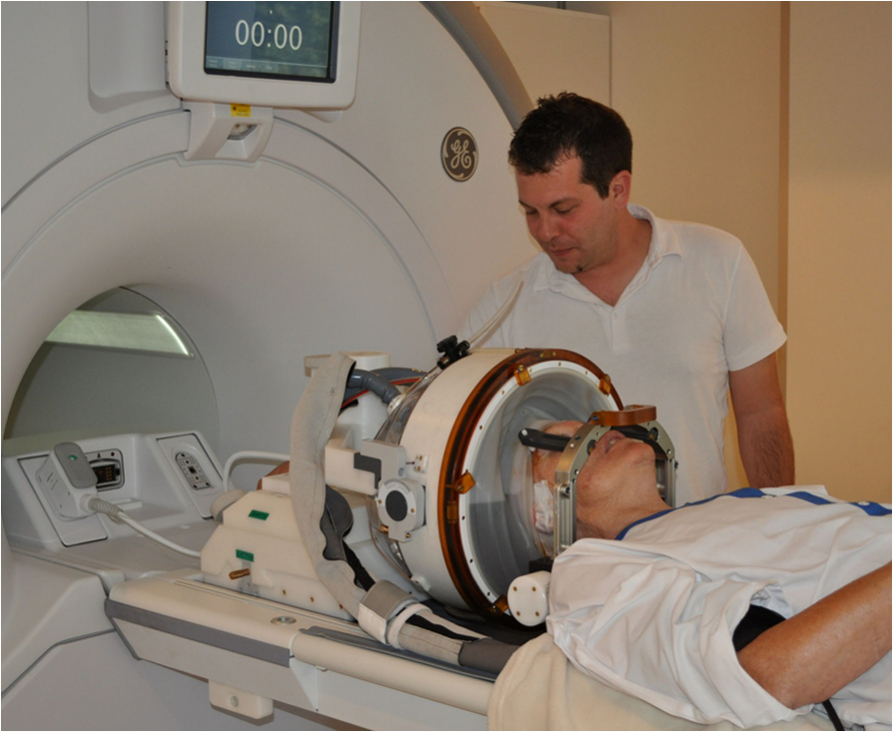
Intra-operative patient setup. Patient lies on MR bed with the head immobilized by a metal frame and placed inside the phased-array ultrasound transducer. The space between the head and the transducer has been sealed by a silicone membrane and is being filled with cooled and degassed water.
